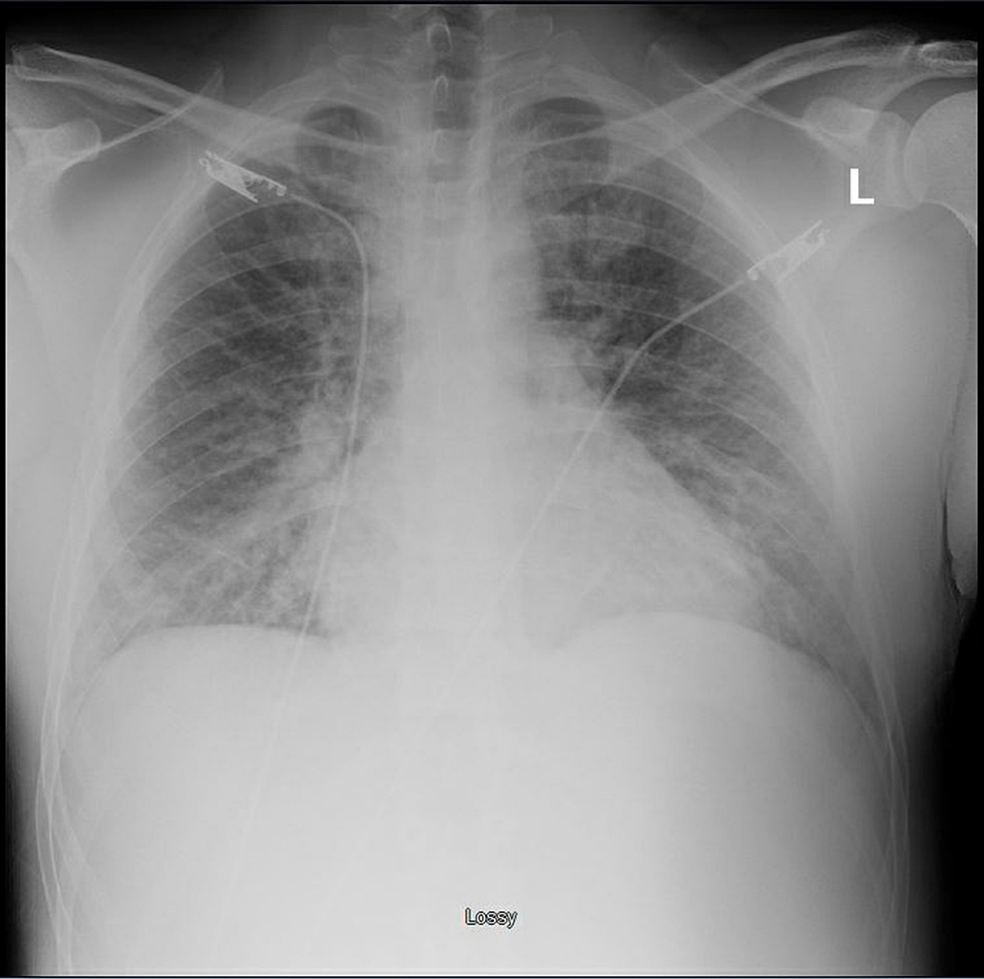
A Chest X-ray on admission (Hospital Day 1) demonstrating pulmonary vascular congestion
